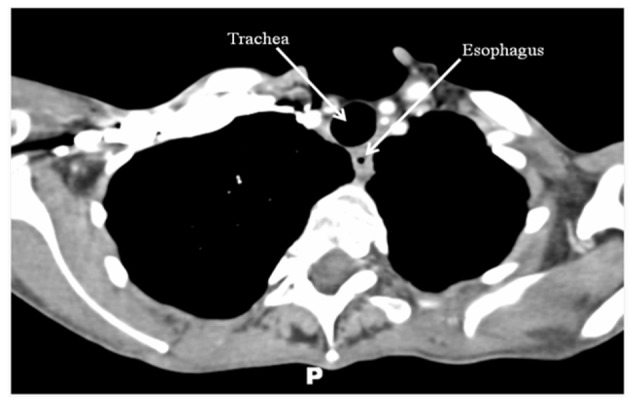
CT scan of the neck/chest with intravenous contrast performed 24 months after treatment demonstrating complete resolution of the thyroid mass and no abnormal lymphadenopathy.
